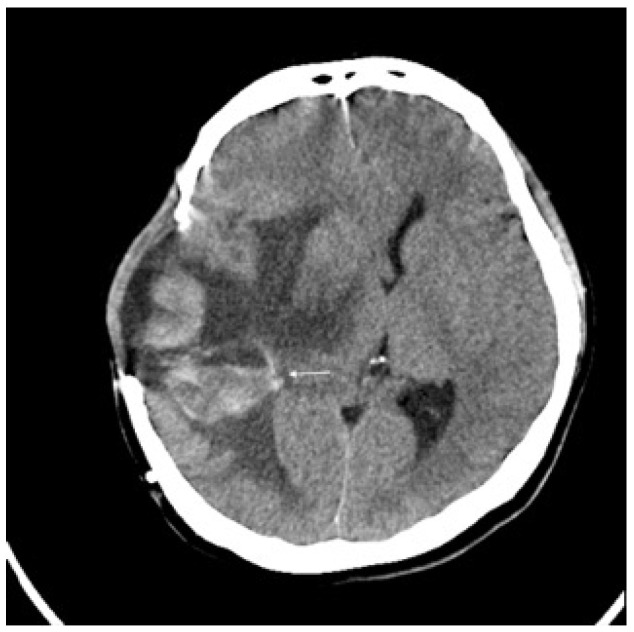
Brain CT without contrast showed recurrent tumor (arrow) with acute hemorrhage.
